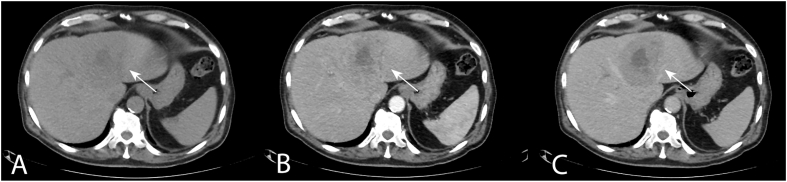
Abdominal CT scan, axial slices. A tumour mass visible in segment 4 invading a small portion of segment 2 of the liver (white arrow). Radiological features are typical for HCC: early contrast enhancement and early wash-out with a non-enhancing central scar.
